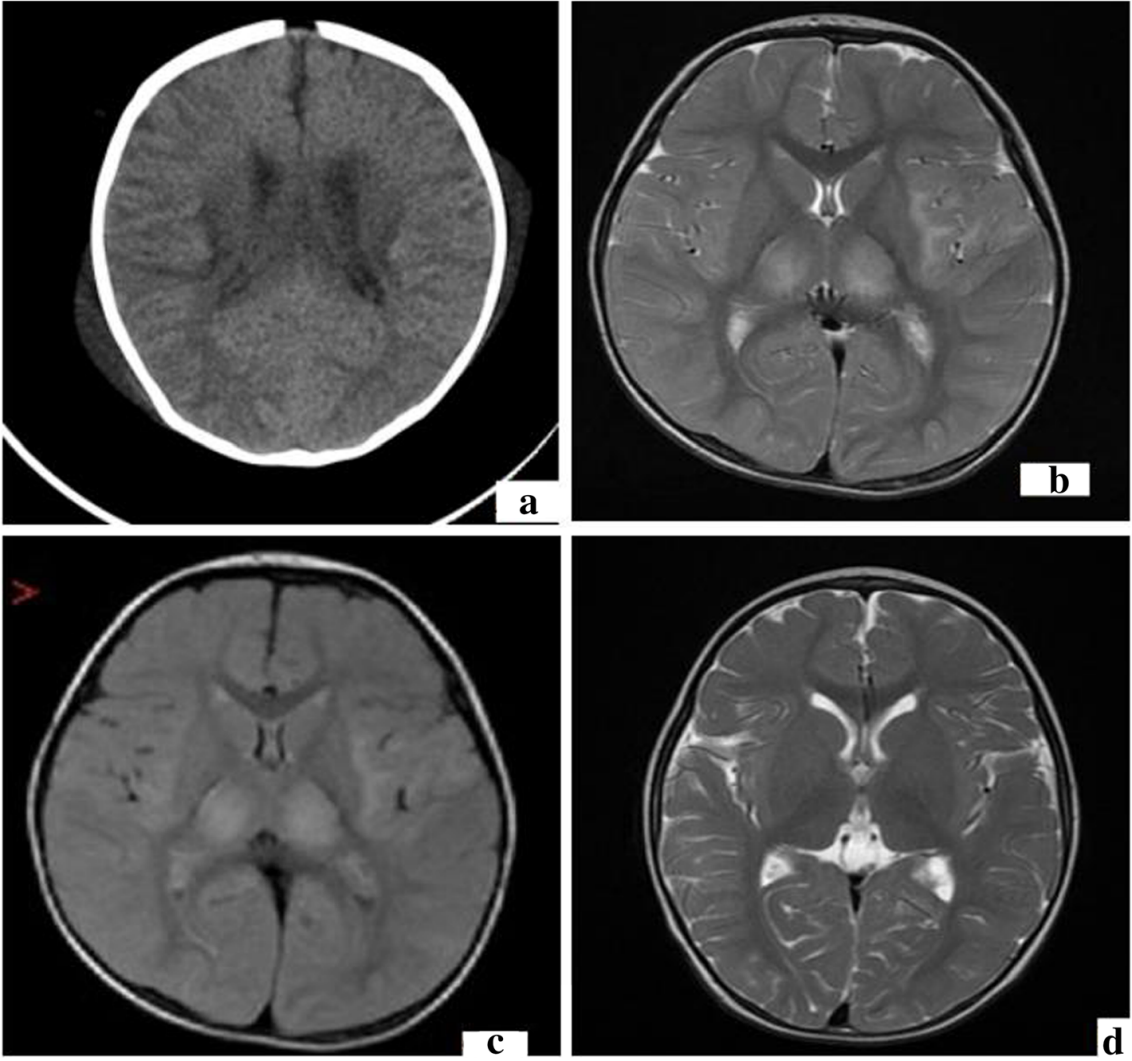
Neurological images. Brain magnetic resonance imaging (MRI) on admission revealed extensive edema (a); after 4 weeks, it showed long T2 signal (b) and hyperintensity on fluid-attenuated inversion recovery (FLAIR, c) mainly in thalamus, basal ganglia, brainstem. 1 year later, the neurological image (MRI) showed slightly abnormal shape of bi-lateral ventricular, not any other obvious abnormal changes noticed (d)
